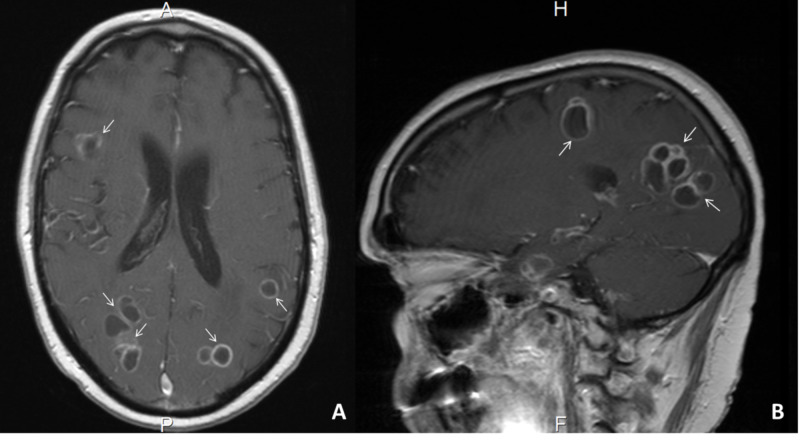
Neuroimaging studies T1-weighted MRI of the Brain (A - axial and B - sagittal views). Slices show multiple multifocal and well-circumscribed supratentorial lesions with restricted diffusion, vasogenic edema and focal mass effect with budding daughter lesions.
